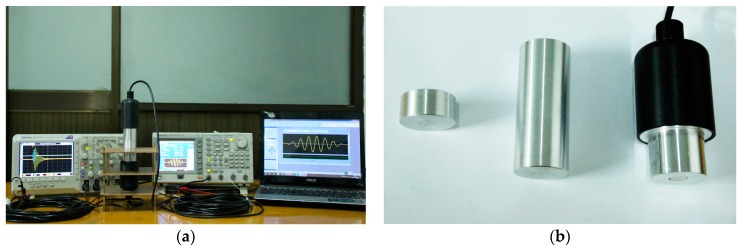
The pitch-catch experimental platform, (a) experimental testing; (b) Aluminum cylinders and sandwiched piezoelectric ultrasonic transducers.
